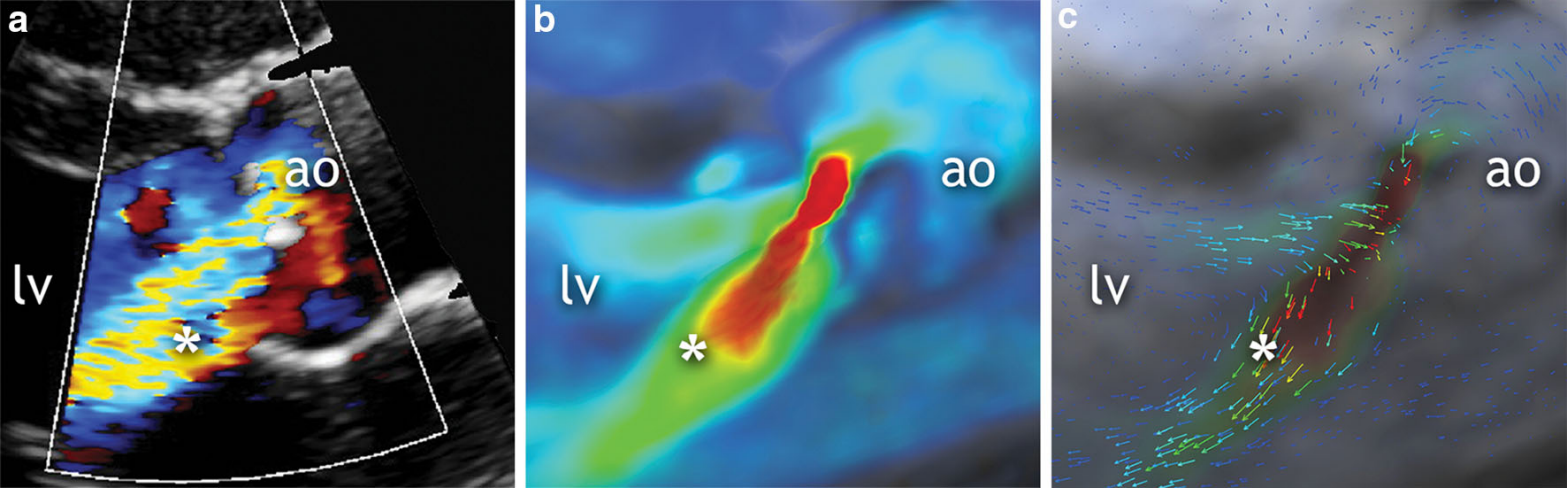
Aortic regurgitation by echocardiography and CMR 4D flow imaging. Case example of a 19-year-old man after balloon dilatation of the aortic valve for congenital aortic stenosis. Panel a Parasternal long axis view of aortic valve in diastole, showing moderate regurgitation (asterisk) demonstrated with color-flow Doppler echocardiography. Panels b and c Corresponding CMR 4D flow images showing the moderate aortic regurgitation. *Asterisk* regurgitant jet, *lv* left ventricle, *ao* aorta
